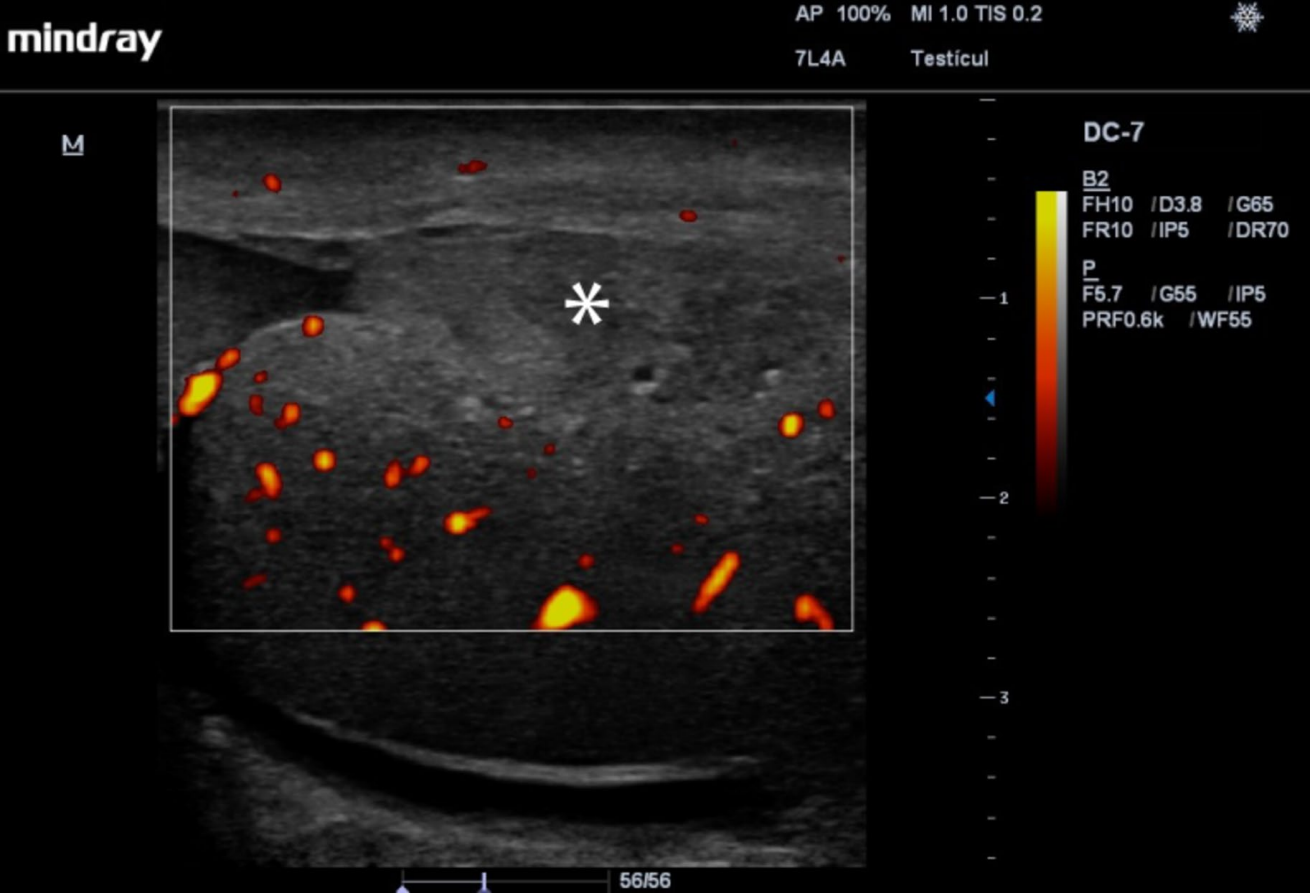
Testicular rupture with interruption of the tunica albuginea, extrusion of the testicular parenchyma (asterisk) through the point of disruption of the same, and a heterogeneous testicular parenchyma. The Power Doppler evaluation shows the absence of vascularization in the affected territory
